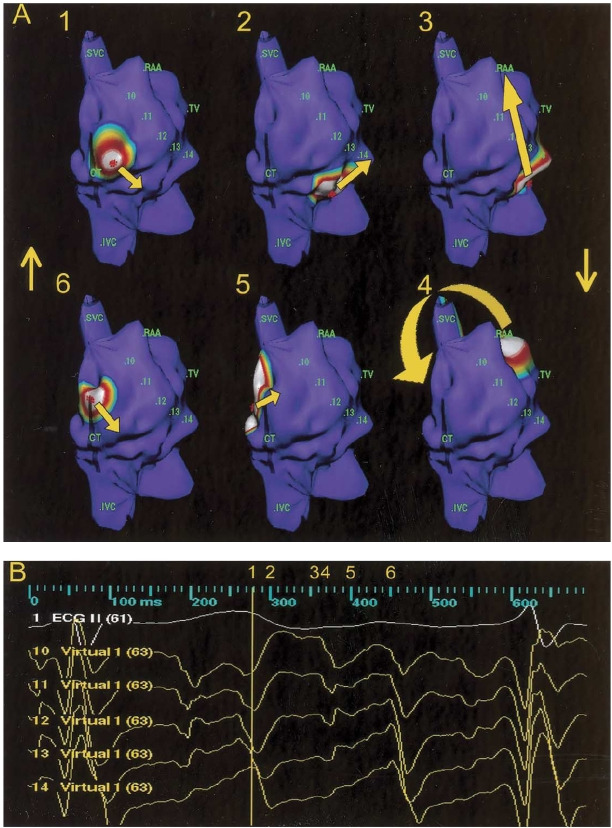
Isopotential maps showing the activation sequence (frame 1 to 6) of single loop reentry in the right lateral view. Color scale for each isopotential map has been set so that white indicates most negative potential and blue indicates least negative potential. The activation wave front proceeds through the channel between the CT and the central obstacle (frame 1), activates the low anterior wall (frame 2), and turn around the line of block (frame 3), then the wave front propagates upward to the roof in front of the right atrial appendage (frame 4), turns around the superior vena cava (SVC) to activate the posterior wall (frame 5), and spreads over the top of the crista terminalis (CT) to complete the reentrant circuit (frame 6). The virtual electrograms (virtual 10 to 14) on the line of block showed double potentials (Reproduced from Tai CT, Liu TY, Lee PC, Lin YJ, Chang MS, Chen SA. Noncontact mapping to guide radiofrequency ablation of atypical right atrial flutter. J Am Coll Cardiol 2004;44:1080-1086, with permission from the American College of Cardiology Foundation.) HIS = his bundle region; IVC = inferior vena cava; RAA = right atrial appendage; TV = tricuspid valve.
